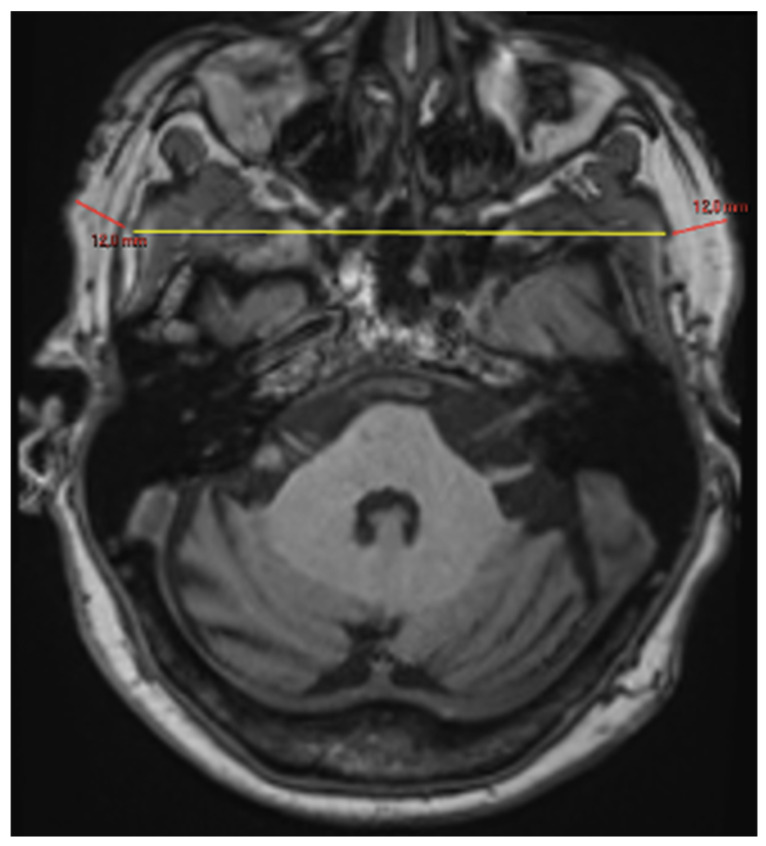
Measurement of Subcutaneous Fat Thickness (SFT). In order to ensure reproducibility, measurements of SFT (mm) are performed on axial T1-weighted sequences in the axial plane using a simple measurement tool on the PACS system, as the maximum perpendicular distance between the fascia and skin, found in the axial plane.
